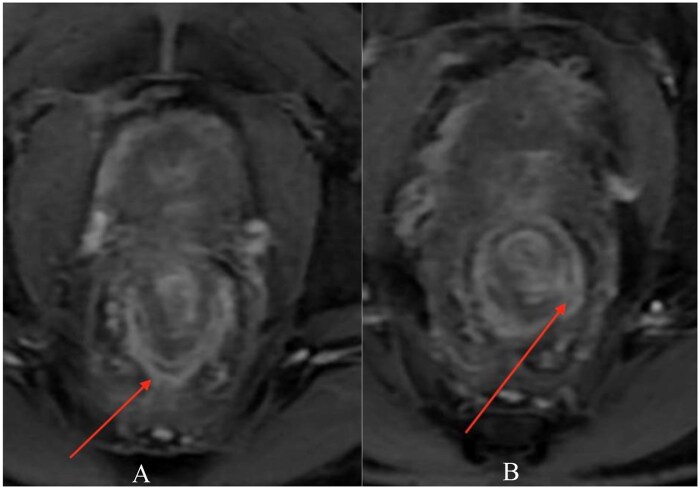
Post-contrast depiction of subtle rectal communication and circumferential perirectal enhancement. (A) Coronal post-contrast fat-suppressed T1-weighted image shows peripheral enhancement around the circumferential perirectal collection, compatible with inflammatory abscess wall/granulation tissue (arrow). (B) Coronal post-contrast fat-suppressed T1-weighted image demonstrates a subtle suspected communication between the rectal lumen and the perirectal collection that is better appreciated after contrast administration (arrow).
